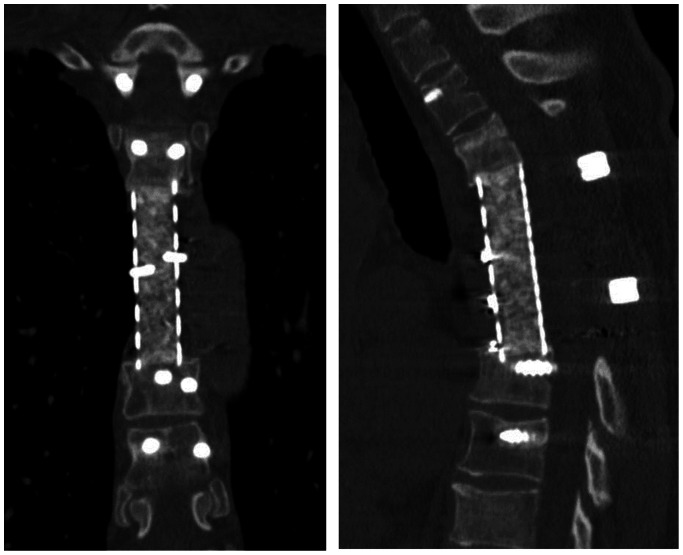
Postoperative computed tomography of the thoracic spine (Left; frontal view, Right; lateral view) one year after TES. Bony fusion can be seen inside the cage.
